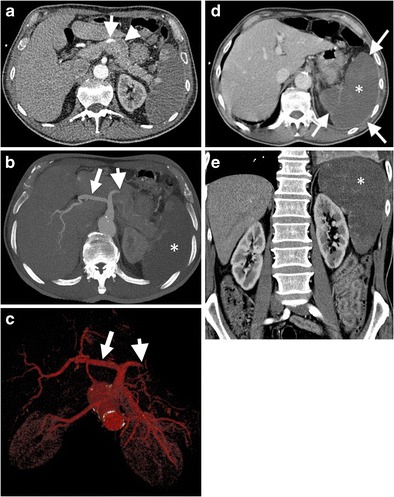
Splenic artery thrombosis. (a) Axial contrast-enhanced arterial phase CT of a 74-year-old man with atrial fibrillation demonstrates patent (*arrow*) and thrombosed (*arrowhead*) portions of the splenic artery. (b) MIP and (c) VR CT images more clearly demonstrate thrombosed splenic (*arrowhead*) and patent hepatic (*arrow*) arteries. (d) Axial and (e) coronal contrast-enhanced CT images at venous phase reveal diffuse splenic infarct (*asterisks*) resulting from splenic artery thrombosis. Capsular enhancement is seen around the spleen (*arrows in d*)
